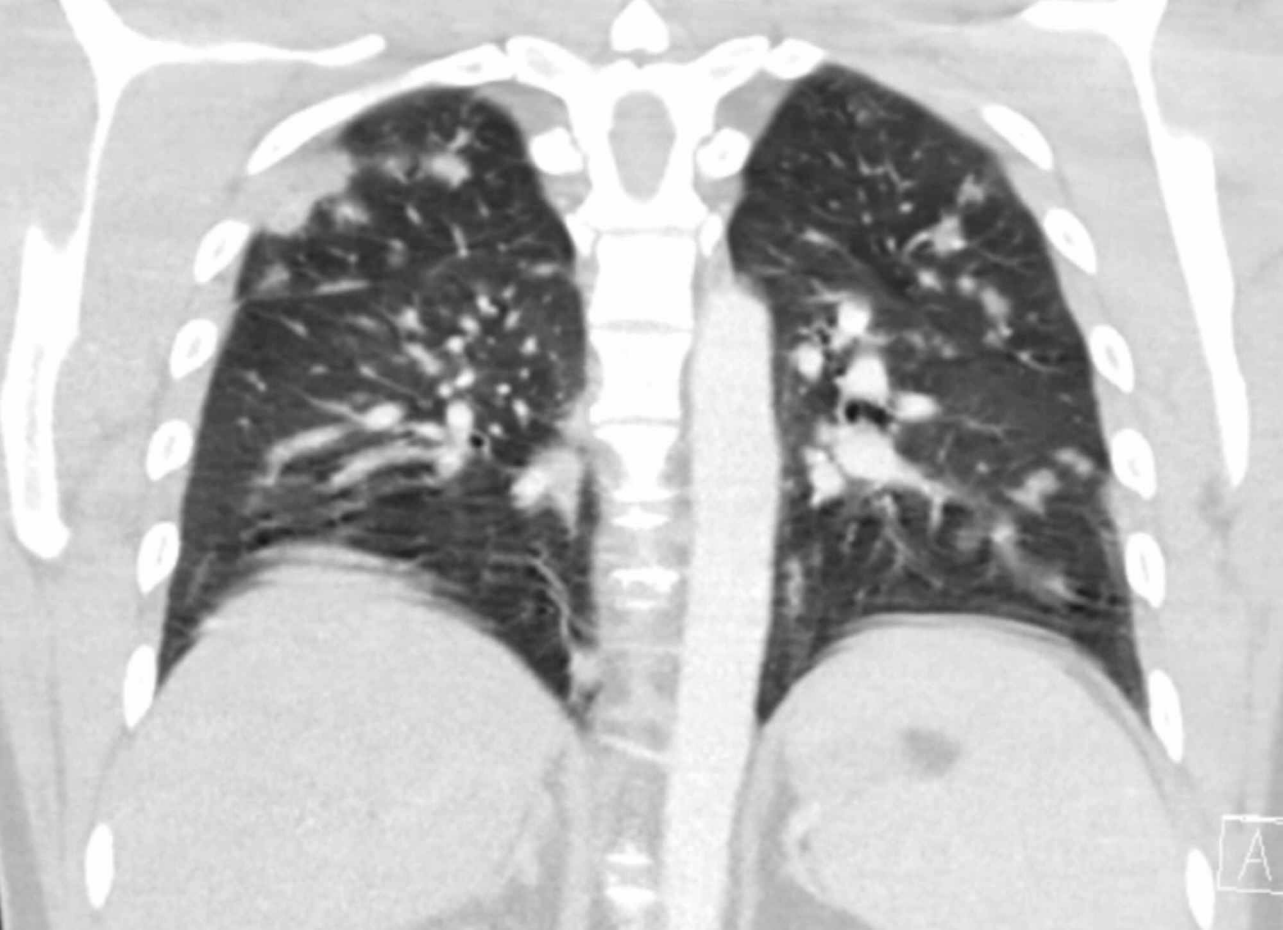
Coronal computed tomography (CT) image of chest showing bilateral ground-glass opacities
